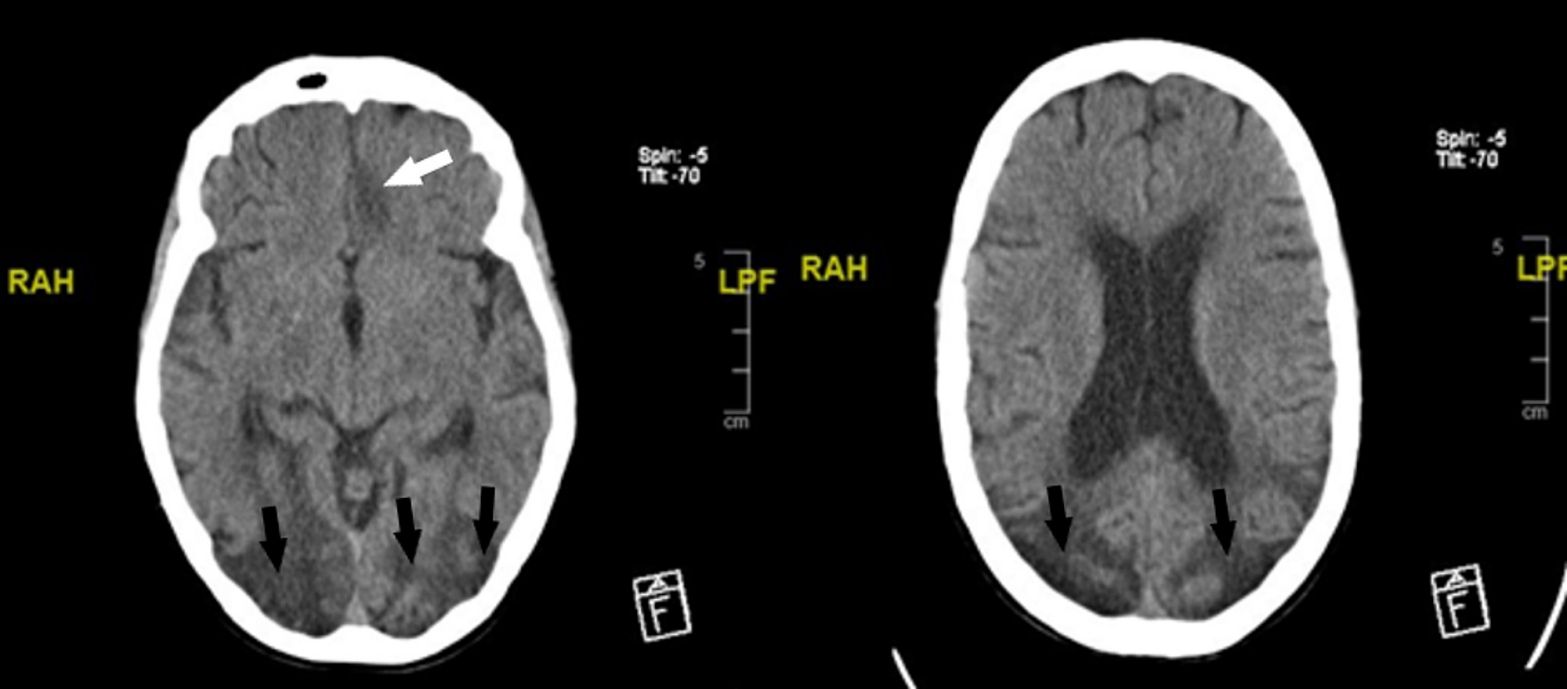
3-mm axial CT head showing bilateral parieto-occipital chronic extensive confluent low attenuation areas are more hypodense and mildly more expanded, likely due to progression of the atrophic changes (black arrows). In the left frontal lobe there is a paramedian moderately hypodense area (white arrow).
